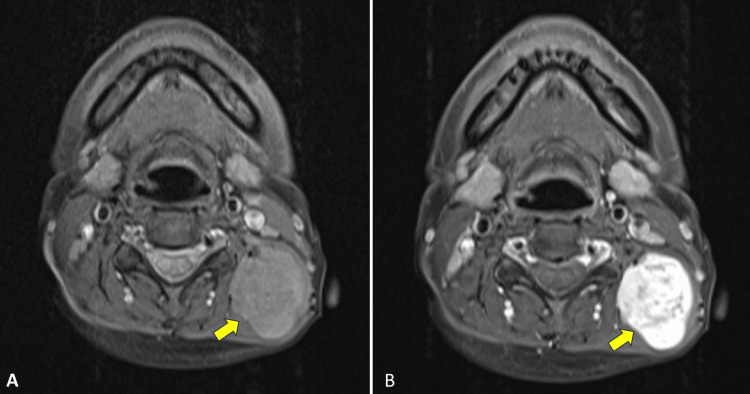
(A) The T1 MR image showing a lobulated mass with intermediate signal intensity within the left prevertebral space measuring 3.5 x 3.3 x 4.4 cm (yellow arrowhead), and (B) the mass shows enhancement after gadolinium contrast administration (yellow arrowhead)
